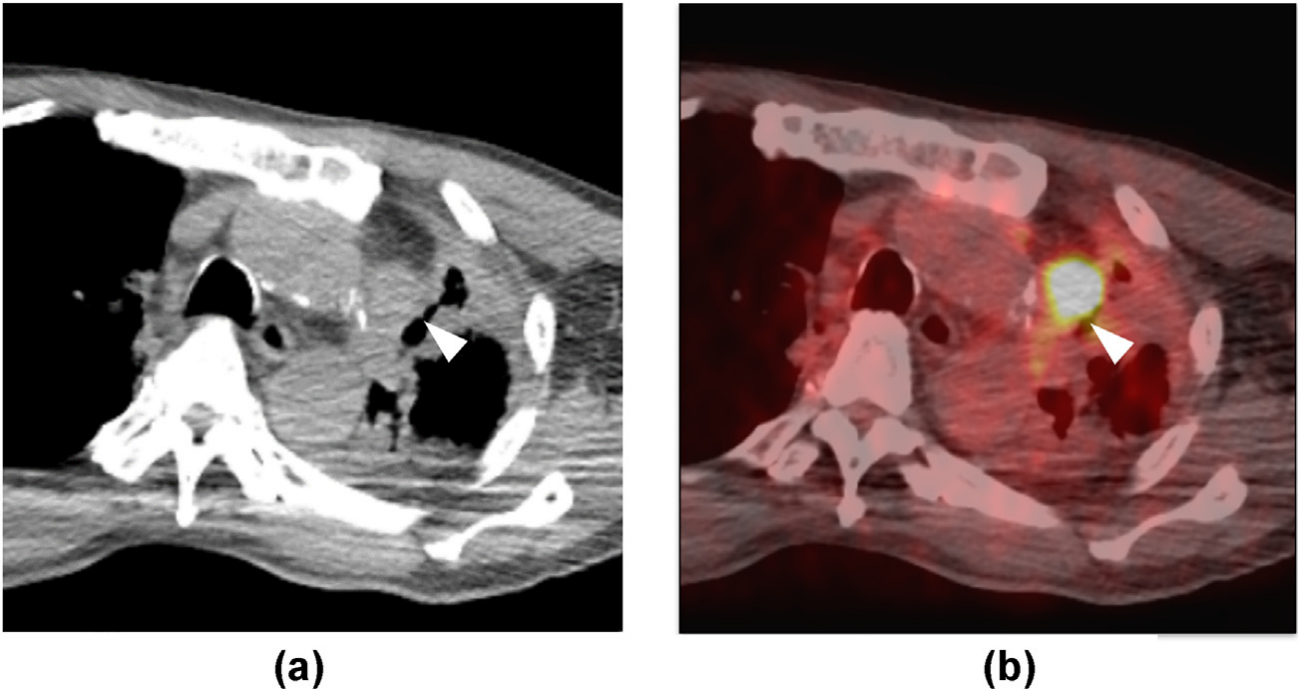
Pretreatment CT image. A nodule 20 mm in diameter (arrowhead) is seen on the mediastinal aspect of the left lung. The lesion abuts the aorta (A). FDG-PET/CT shows radionuclide uptake in the same lesion (B, arrowhead).
